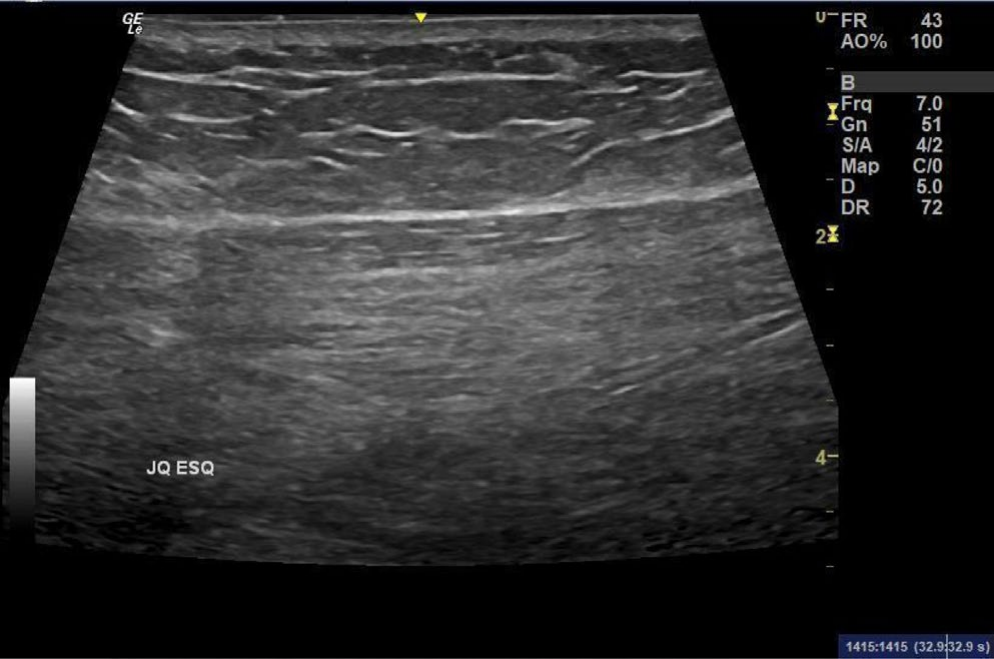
Third polymethylmethacrylate. Ultrasound image (10) showing increased echogenicity and heterogeneity of muscle fibers in the gluteus maximus, characteristic of PMMA injection into the muscular plane.
